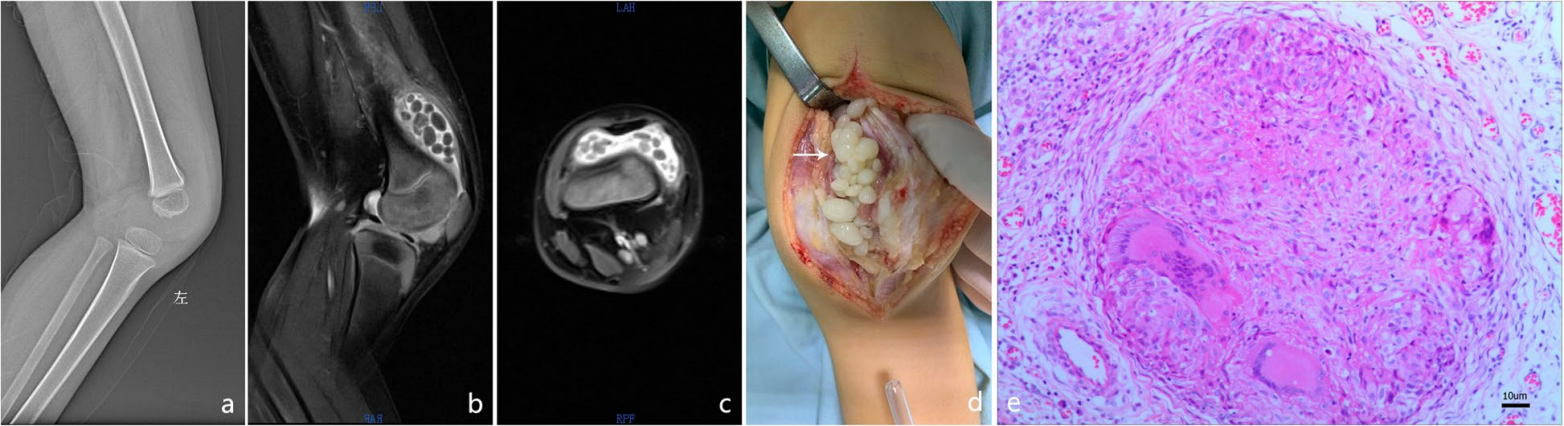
Radiologic, intra-operation and pathology result of left knee joint. a X-ray shows the change of the left knee joint space. b, c MRI revealed abnormal signal changes in the left knee joint. d There were a large number of white translucent round loose bodies (white arrow) overflowing in the joint cavity. e Pathological results shows granulomatous tissue, epithelioid cells and typical Langhans giant cell (black arrow)
